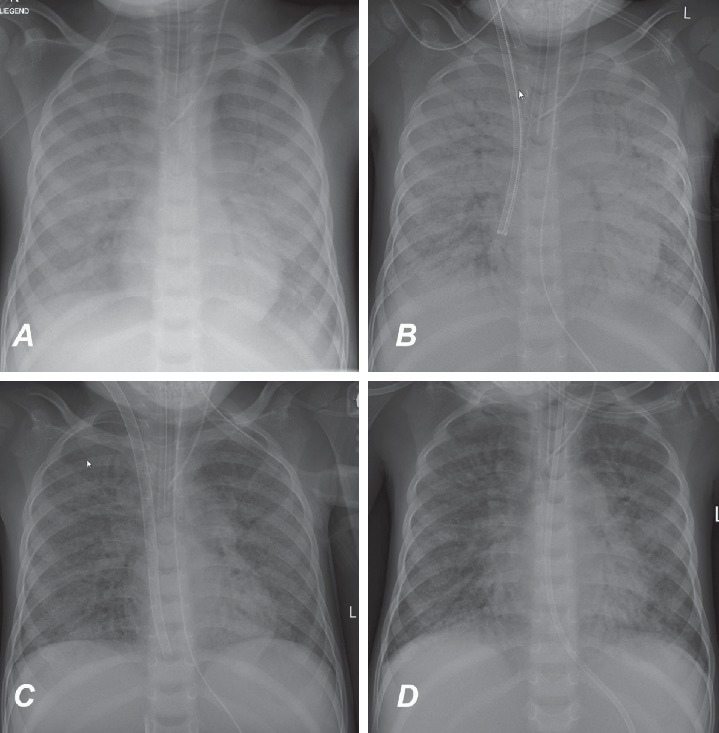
(a) Chest X-ray on admission. (b) Chest X-ray after ECMO cannulation via neck vessels. (c) Chest X-ray after conversion to veno-venous ECMO by using single venous neck cannula. (d) Chest X-ray after ECMO weaning.
